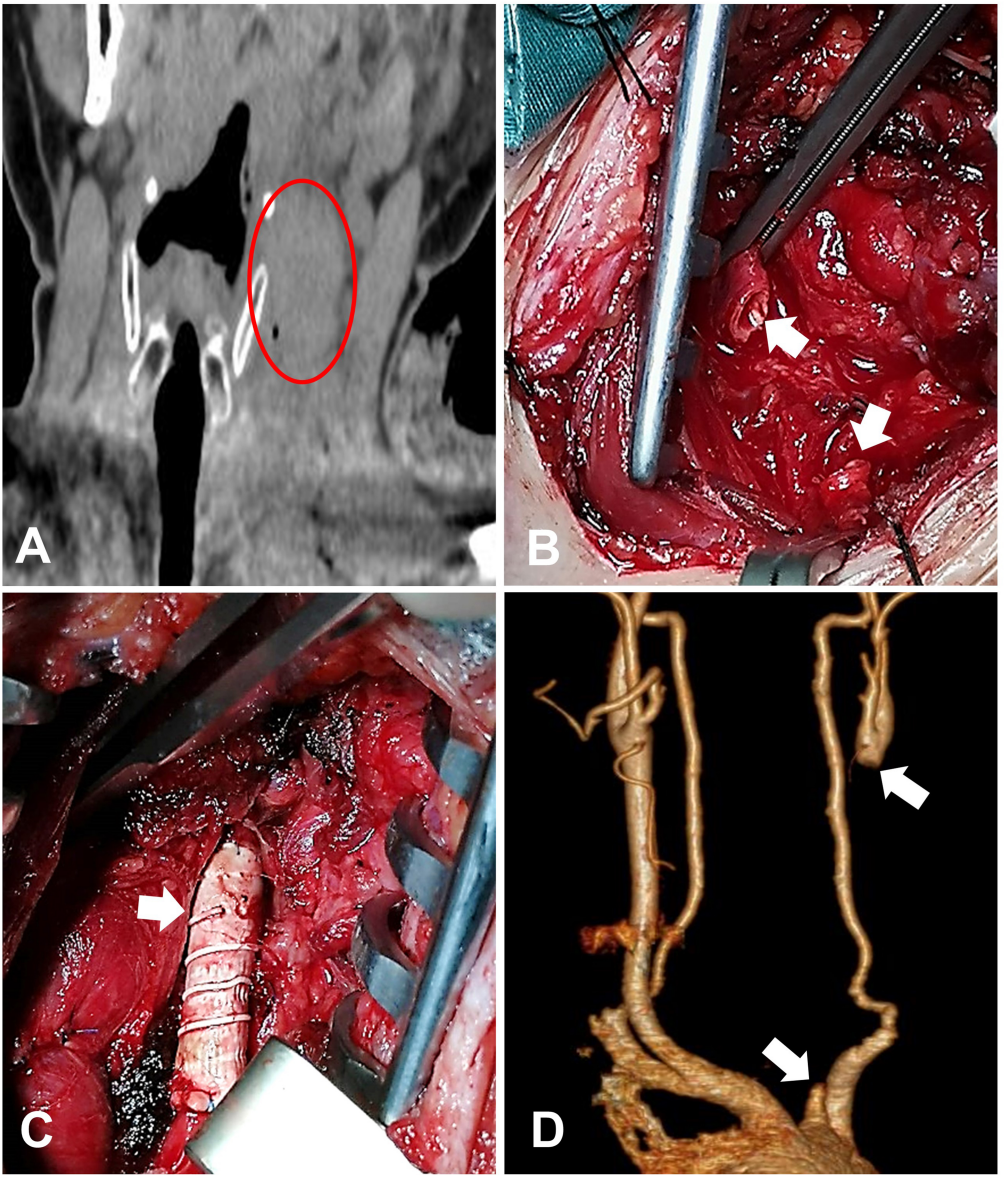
Case 1: (A) coronal CT of the neck showed massive blood clots around the left carotid artery, which compressed and displaced the trachea. Note: the red circle is marked in the left carotid sheath. (B) Intraoperative exploration of left common carotid artery amputation. Note: the arrow is marked as the broken end of the left common carotid artery. (C) Place the image of an artificial vascular anastomosis. Note: the arrow is marked after artificial vascular implantation. (D) Three-dimensional postoperative computer tomographic scan showing a clogged left carotid. Note: the arrow points to the broken end of the left common carotid artery.
